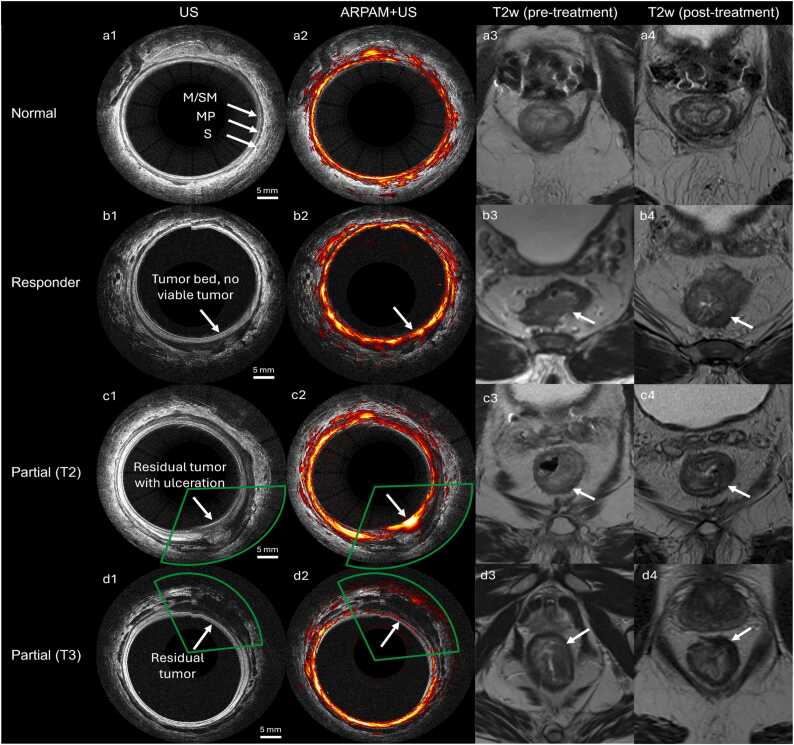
Representative ARPAM-US B-scans and pre- and post-treatment T2w MRI across response categories. Normal rectum shows distinct wall layers on ultrasound (a1) and dense mucosal/submucosal vasculature on photoacoustic imaging (a2). In complete responders, photoacoustic imaging demonstrates restored vascular patterns (b2) in the treated tumor bed. Contrarily, partial responders exhibit disrupted vasculature (c2,d2) and ulceration (c2) on photoacoustic imaging; Notably, the disrupted vascular pattern at the 5-o’clock position (c2) corresponds to the sudden structural change seen on ultrasound (c1). However, these patterns are not differentiated on post treatment MRI (b4, c4, d4), where partial responders showed persistent intermediate T2 signal along the tumor bed without significant change from the pre-treatment exam.
